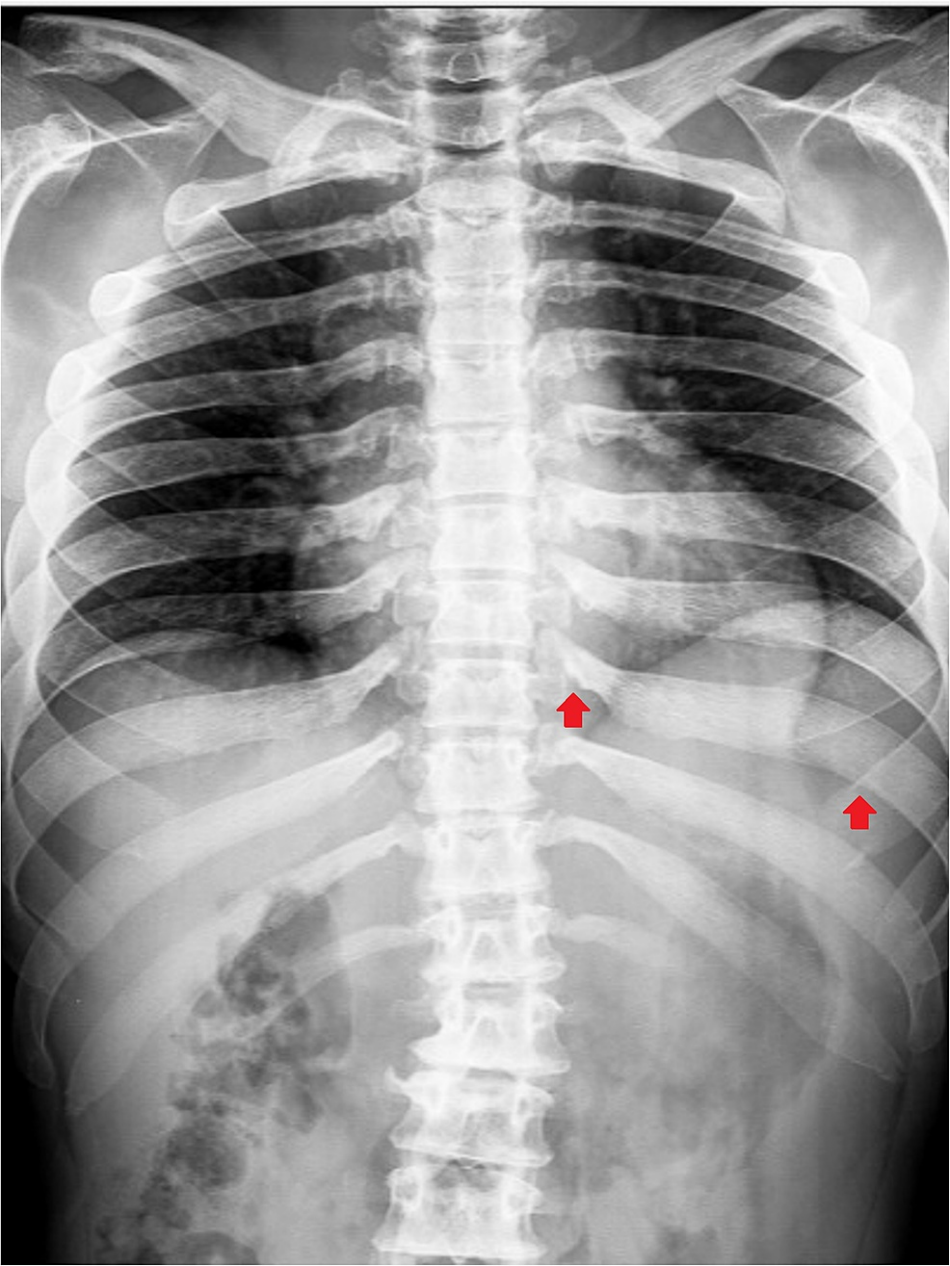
Plain thoracic and abdominal X-ray of our patient showing dysostosis multiplex manifested as ribs broadened distally and narrowed at the takeoff from the vertebral bodies, resulting in the oar shape (red arrow).
